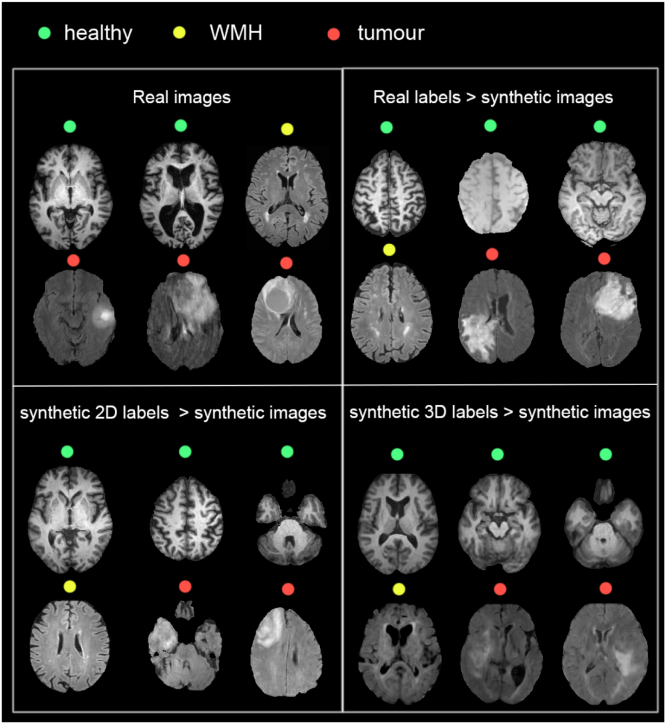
Example healthy and diseased images coming from (top left): real distribution; (top right): images generated from real labels; (bottom left): images generated from synthetic 2D labels; (bottom right): images generated from synthetic 3D labels. T1 was chosen for healthy images to display better tissue contrast, and FLAIR for WMH and tumour images.
